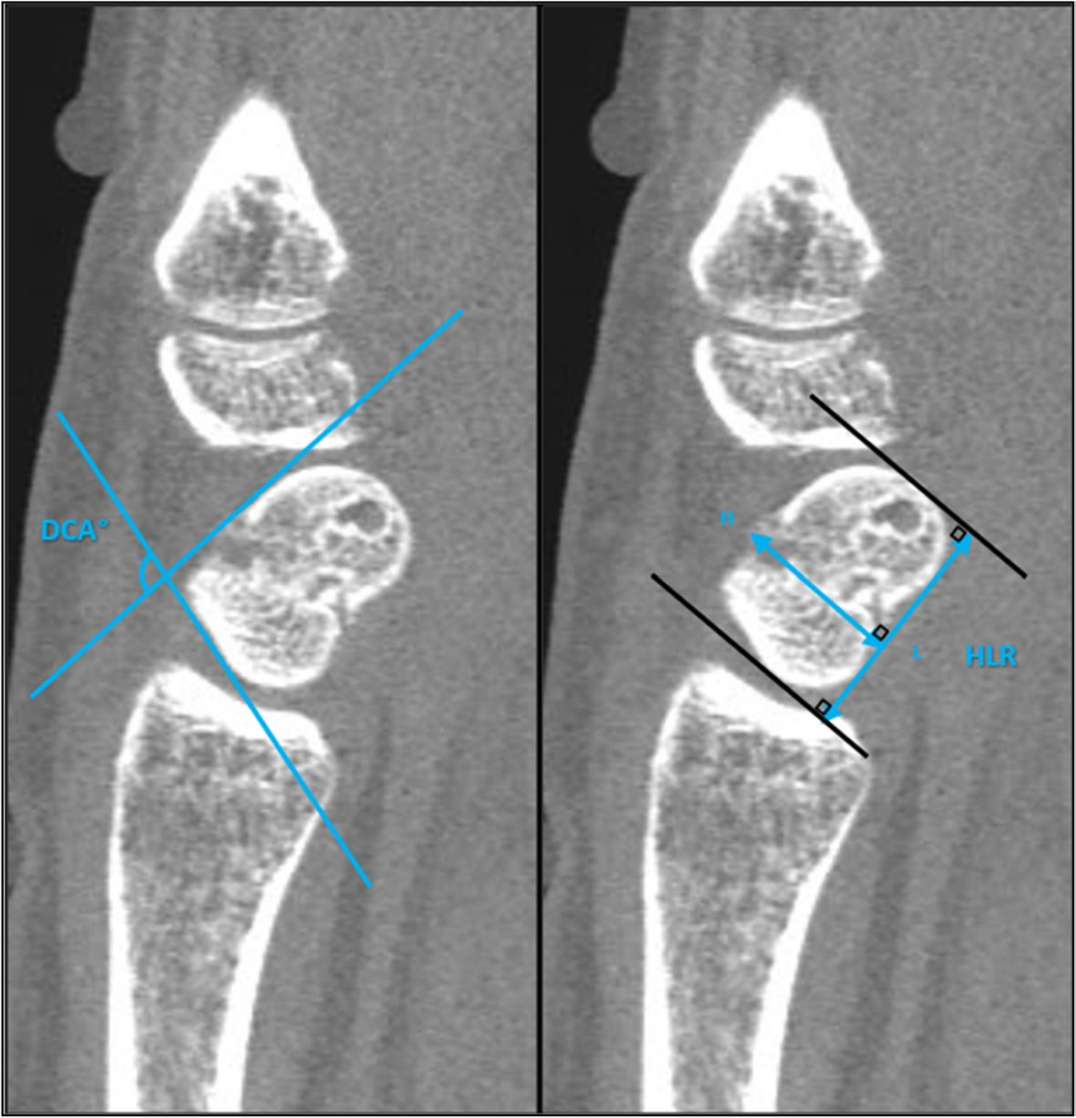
Sagittal CT scan of scaphoid non-union. The measurement of dorsal cortical angle (DCA) and height-length ratio (HLR). The height-length ratio is calculated by dividing the scaphoid height by length
